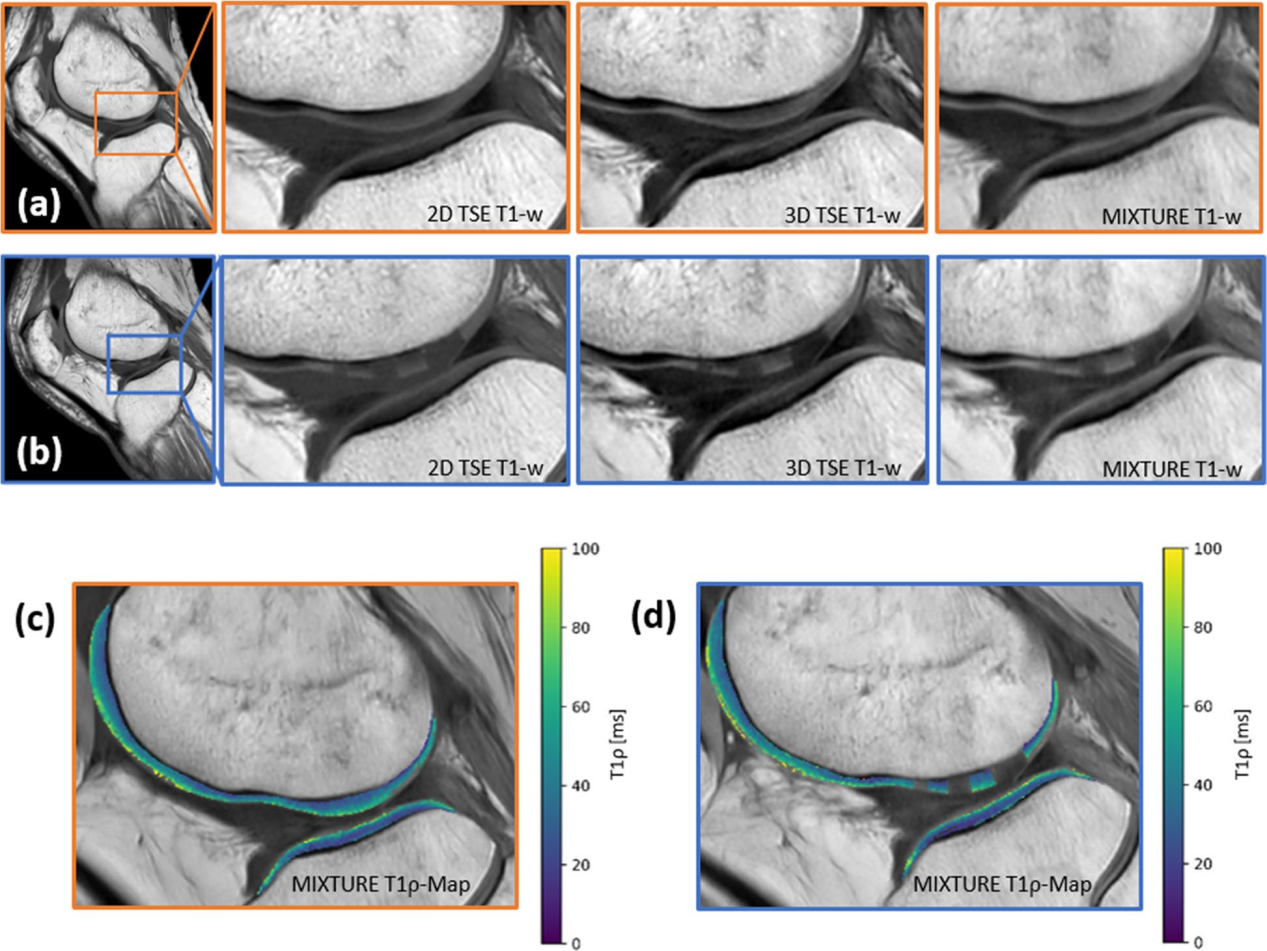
Representative T1-weighted images and MIXTURE T1ρ maps. Morphologic images (a, b) and corresponding MIXTURE-based T1ρ maps (c, d) are visualized before and after defect creation. Figure organization as in Fig. 4. *MIXTURE* Multi-interleaved x-prepared turbo spin-echo with intuitive relaxometry
